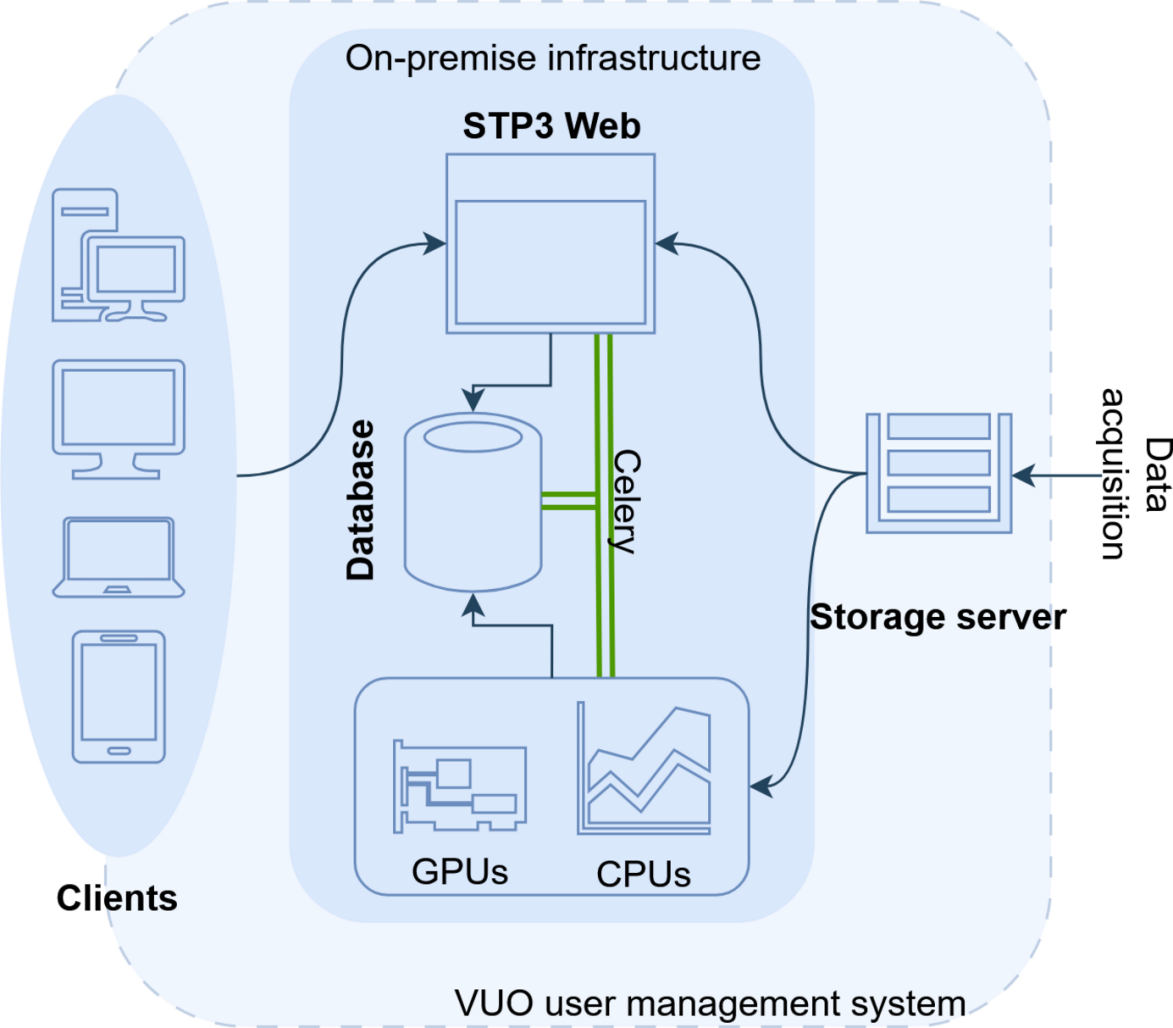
Current use case, *STP3 Web*. This is a CT data processing program which is built by partly reusing existing legacy desktop application code (backend and calculations) and the *MAPI* interface layer with the *Celery* intermediate queue and task management system. This allows each individual end user to use available computing resources directly from a website and ensures robust and secure operations. Legend: green lines (*Celery*) indicate job control/dispatch messages via the *Redis* broker and database pointers, and thin arrows indicate API calls and data paths (HTTP requests between services and file I/O between storage and workers).
